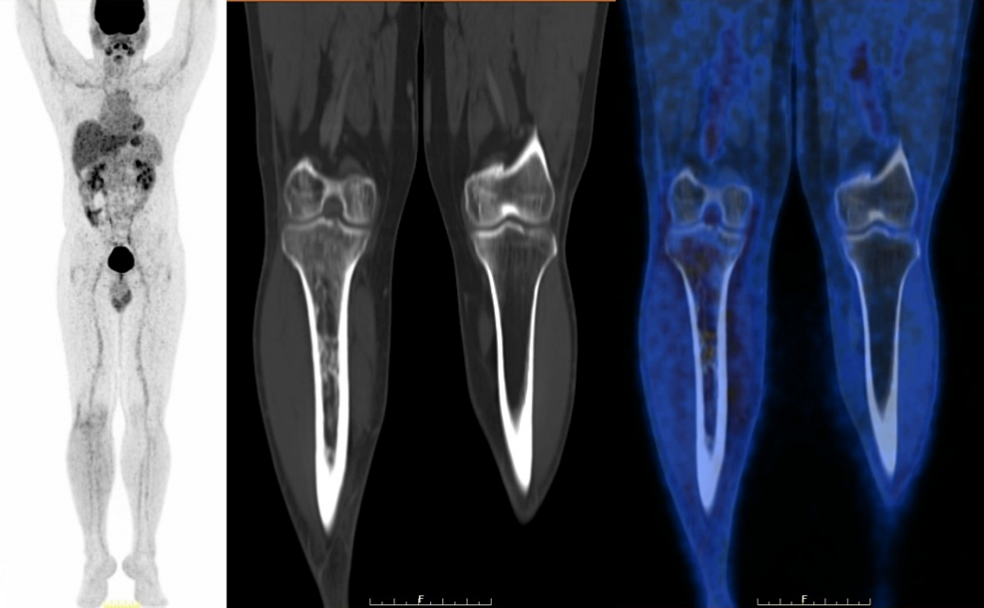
Positron emission tomography, three months after completion of chemotherapy and radiotherapy Metabolic resolution of permeative lytic lesion with intramedullary sclerosis involving diaphysis extending to metaphysis and epiphysis of the right tibia - Status quo in CT, Deauville score 1, post chemo-radiation. Resolution of FDG non-avid lymph nodes over right external iliac, bilateral superficial inguinal, right femoral node and right popliteal fossa were noted.
